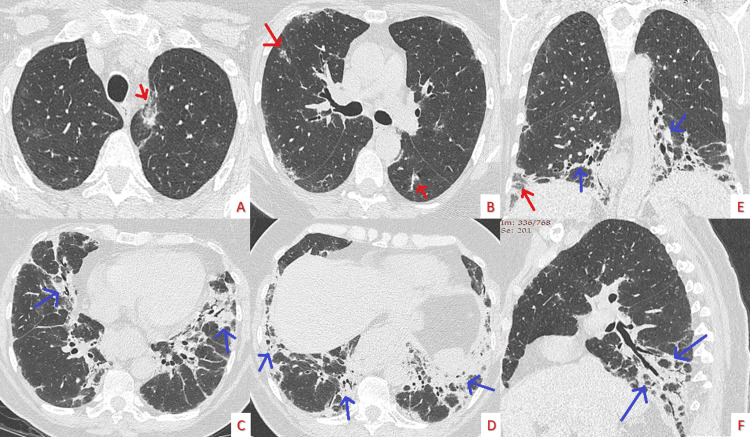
Admission chest computed tomography Bilateral ground glass opacities (red arrows) and patchy consolidations (blue arrows) with a central irregular peribronchovascular and perilobular distribution; axial slices from lung apices to bases (A-D), coronal image (E), sagittal image (F).
